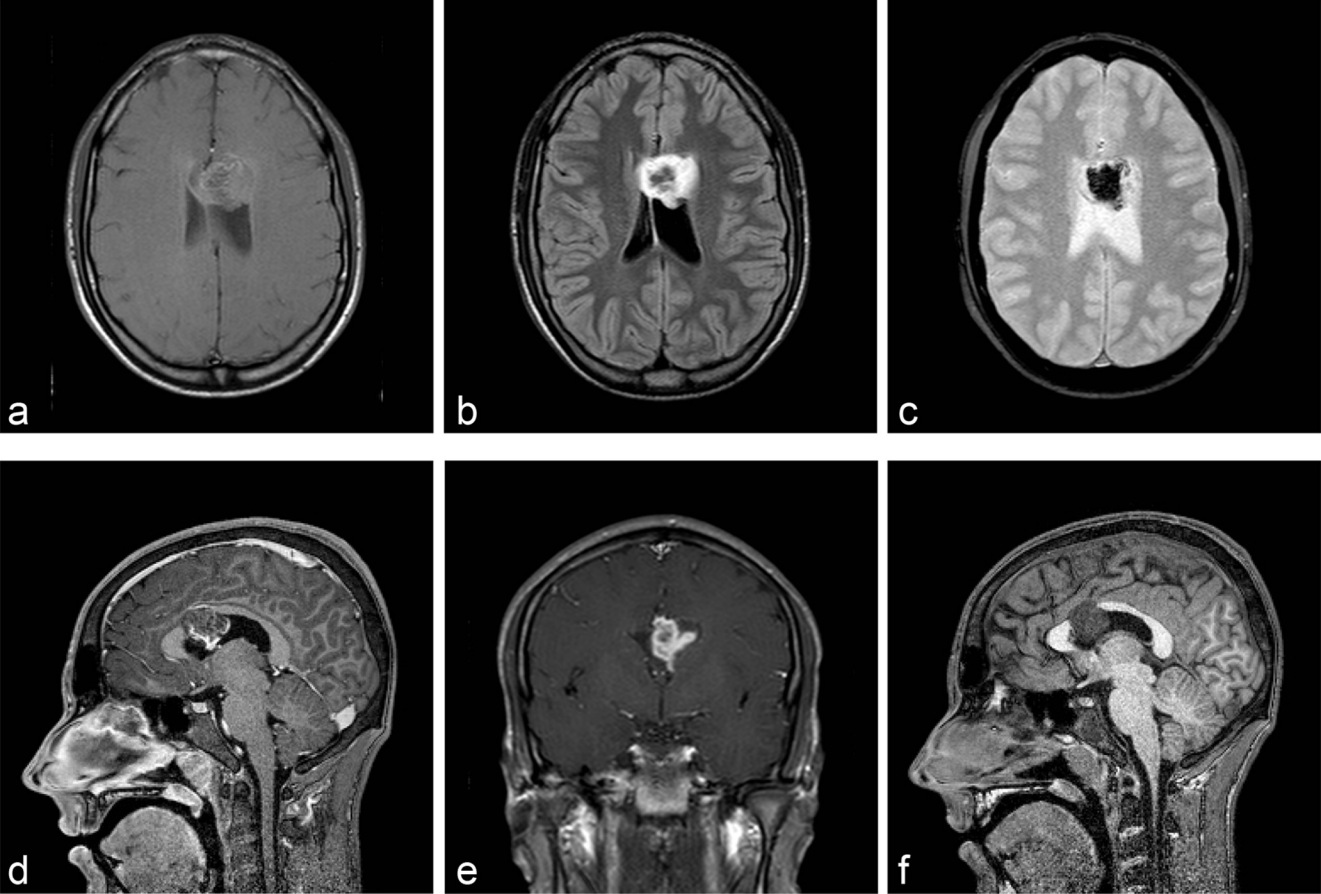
Axial brain MRI scan shows an intraventricular expansive lesion with hypointense signal on *T*_1_ (a) and hyperintense signal on FLAIR (b). Axial gradient echo shows the haemorrhagic component (c). Sagittal contrast-enhanced *T*_1_ weighted shows the lesion enhanced heterogeneously by the gadolinium (d). It occupies predominantly the anterior horn of the left lateral ventricle—coronal contrast-enhanced *T*_1_ (e) and infiltrates the anterior portion of the corpus callosum—sagittal *T*_1_ (f). FLAIR, fluid-attenuated inversion-recovery.
